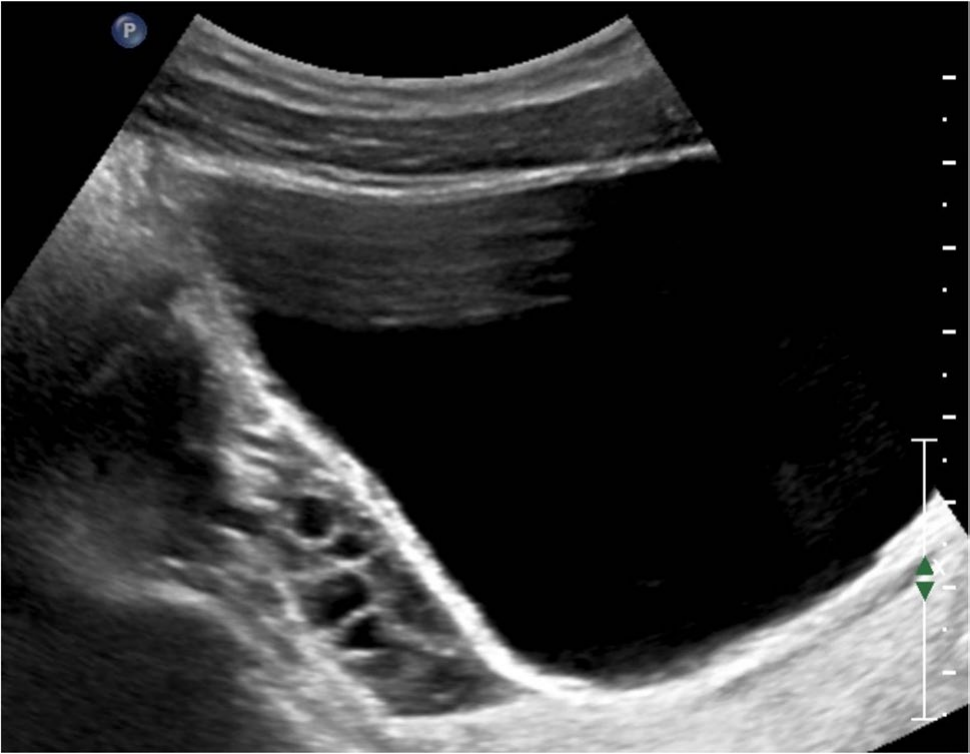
Sagittal gray-scale pelvic ultrasound image of the right ovary in an 11-year-old prepubertal girl with multiple follicles of different sizes
